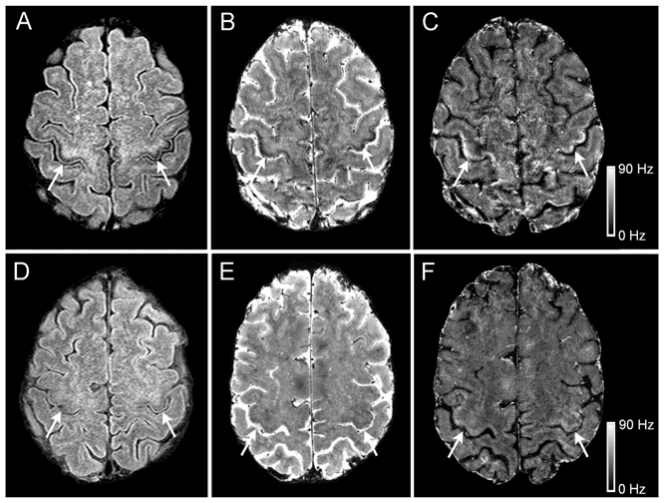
3T and 7T MRI in ALS and healthy control. 3T and 7T brain MRI signal changes in the motor cortex hand knob differed between ALS patients (A, B, C) and healthy controls (D, E, F). Cortical hypointensity (*arrows*) is shown to be present in an ALS patient (Patient 2, age 51) and not in a healthy control on 3T FLAIR (A, D) and 7T gradient echo magnitude images (B, E). These areas corresponded to hyperintensity in the 7T R_2_
